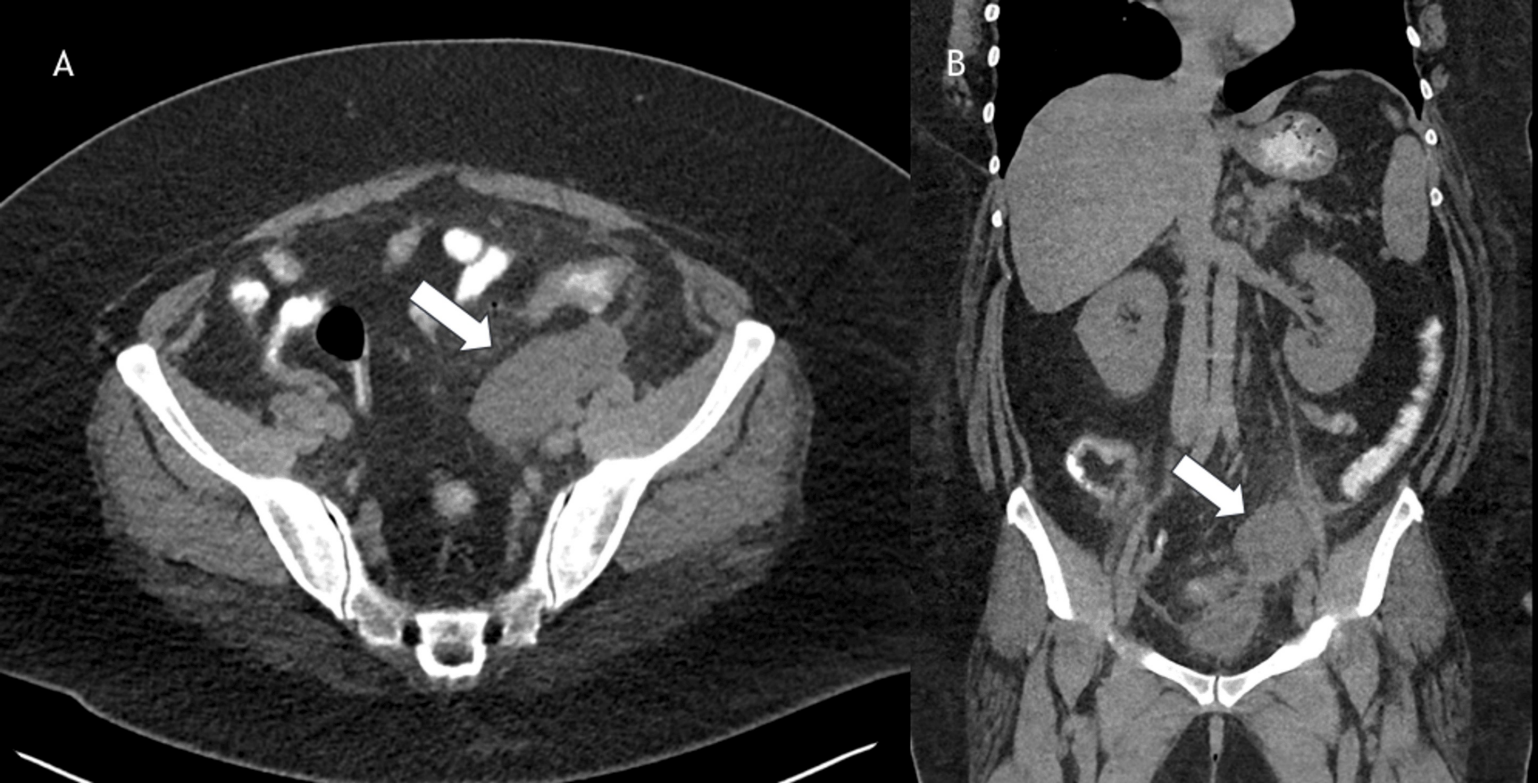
Non-contrast computed tomography (CT) showing left adnexal lesion with surrounding fat stranding (1A) Transverse view, (1B) Coronal view.
